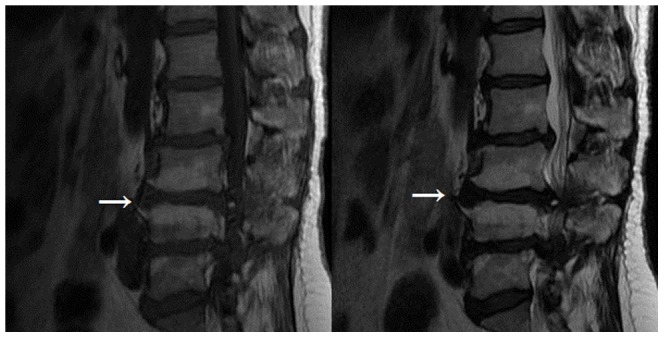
Modic type II changes (indicated by arrow) appear hyperintense on. T1-weighted and isointense or hyperintense, on T 2 weighted images.
